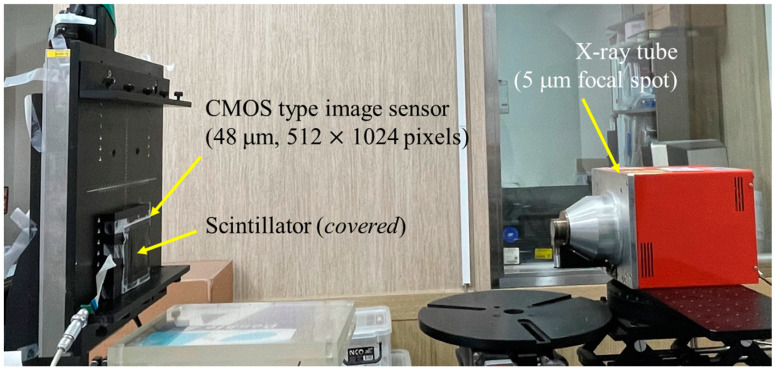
Photographs of the radiography imaging system, which consists of an X-ray tube (L10321, Hamamatsu Photonics K.K., Japan focal spot size: 5 μm) and a CMOS type image sensor (Rad-icon, pixel size: 48 μm, pixel matrix: 512 × 1024 pixels, and analog-to-digital conversion resolution: 16 bit) used in the experiment. CMOS, complementary metal–oxide–semiconductor.
